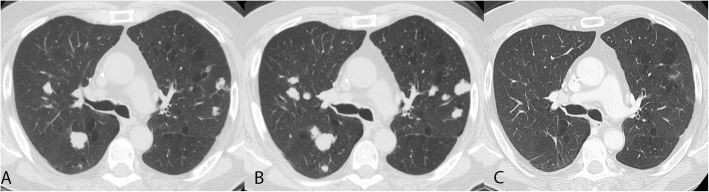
Computerized tomography (CT) images of lung nodules. CT at time of initiation of nivolumab (a), 2 months after initiation of nivolumab (b) and 6 months after initiation of nivolumab (c)
